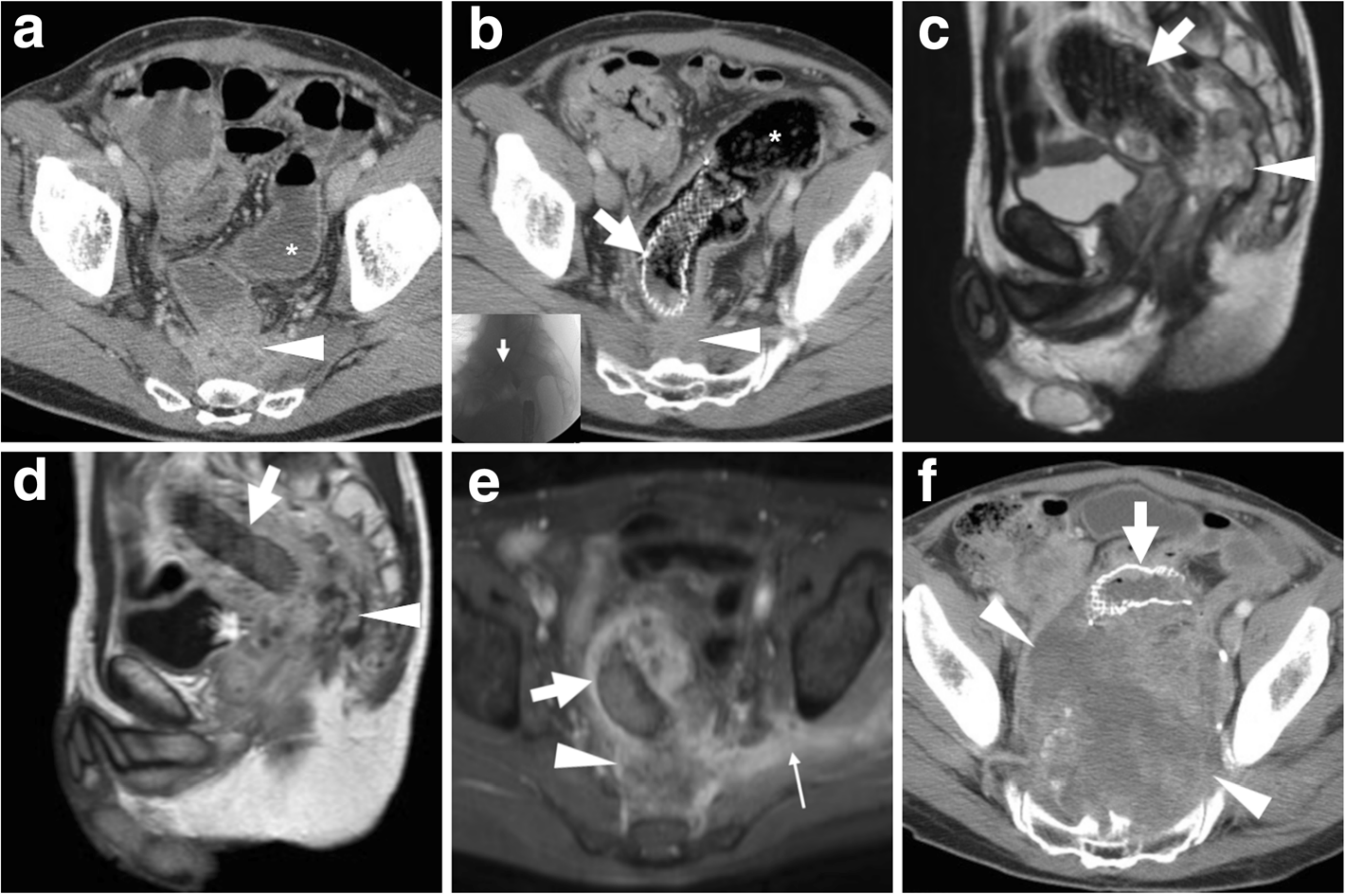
Stent management of an extracolonic pelvic tumour and neoplastic outgrowth. After diagnosis of local recurrence (arrowheads in CT, a) of previously resected rectal cancer causing upstream bowel obstruction (*), palliative positioning of a SEMS (thick arrows) was performed (post-procedural CT (b), intraprocedural fluoroscopy in inset). Despite intensive chemotherapy, follow-up MRI including sagittal T2- (c), post-gadolinium sagittal (d) and fat-suppressed axial (e) T1-weighted images confirmed patent SEMS (thick arrows) and progressive inhomogeneity of tumour mass (arrowheads) consistent with abscess formation and development of fistulisation through the right ischiatic foramen (thin arrow in e). The neoplastic recurrence (arrowheads in CT, f) ultimately grew to massive size causing ventral dislocation and compression of the SEMS (thick arrow)
